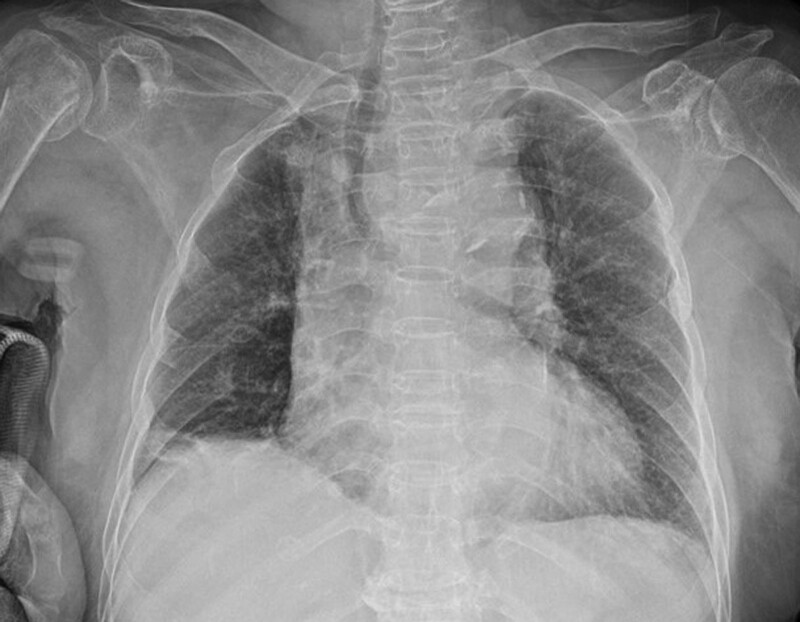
Chest radiography. Trachea is deviated to the right.
